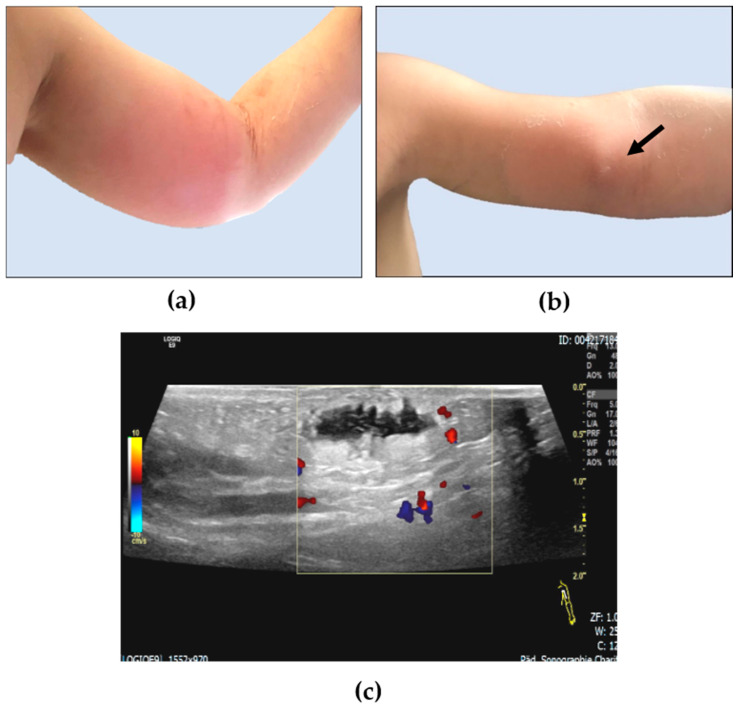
Photographic documentation of dinutuximab beta extravasation in the soft tissue of the left upper arm in a 3-year-old patient on the day of diagnosis (a) and on day 6 (b): a palpable lesion on day 6 (black arrow) was further investigated by sonography, which demonstrated an anechoic non-vascularized liquid encapsulation of 2 mL volume surrounded by panniculitis (c).
